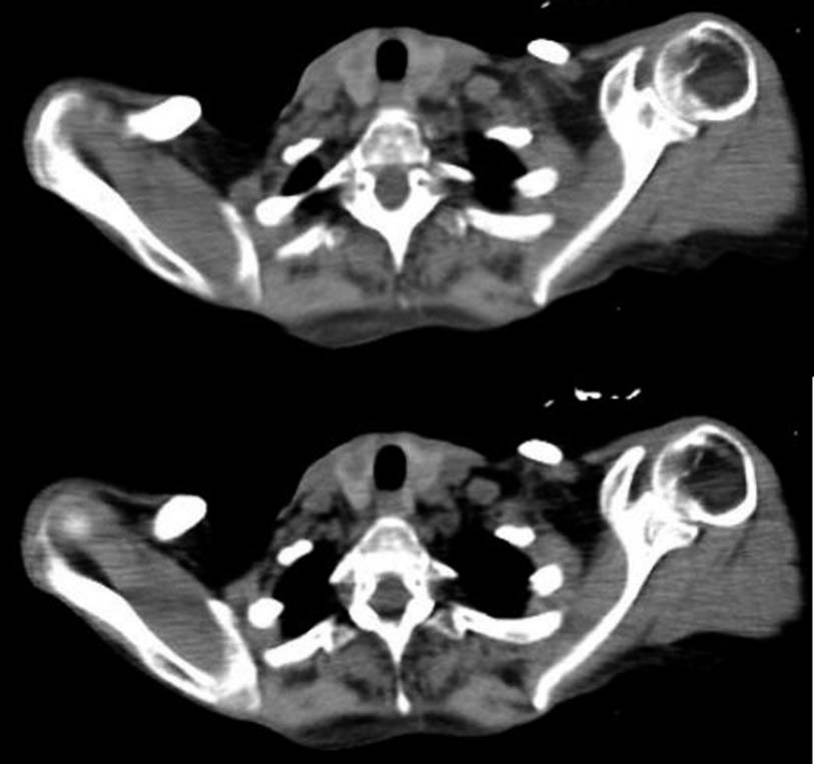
A CT scan, showing the multinodular appearance of the thyroid gland. CT, computed tomography.
